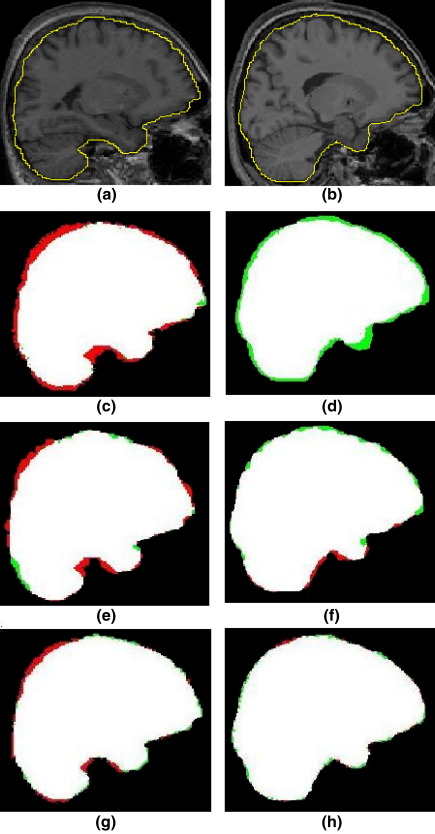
Automated methods error on an MRI sagittal slice of a typical subject imaged at 1.5T (left) and 3T (right). Areas of negative error (estimate smaller than the manual reference) are shown in green, areas of positive error in red. Areas identified as intracranial by both the gold standard and the automated method segment are shown in white. Top panel: MR images with isolines of the manual delineation. Second panel: SPM-tissue class method (default setting) output. Third panel: BET output. Bottom panel: RBM output.
